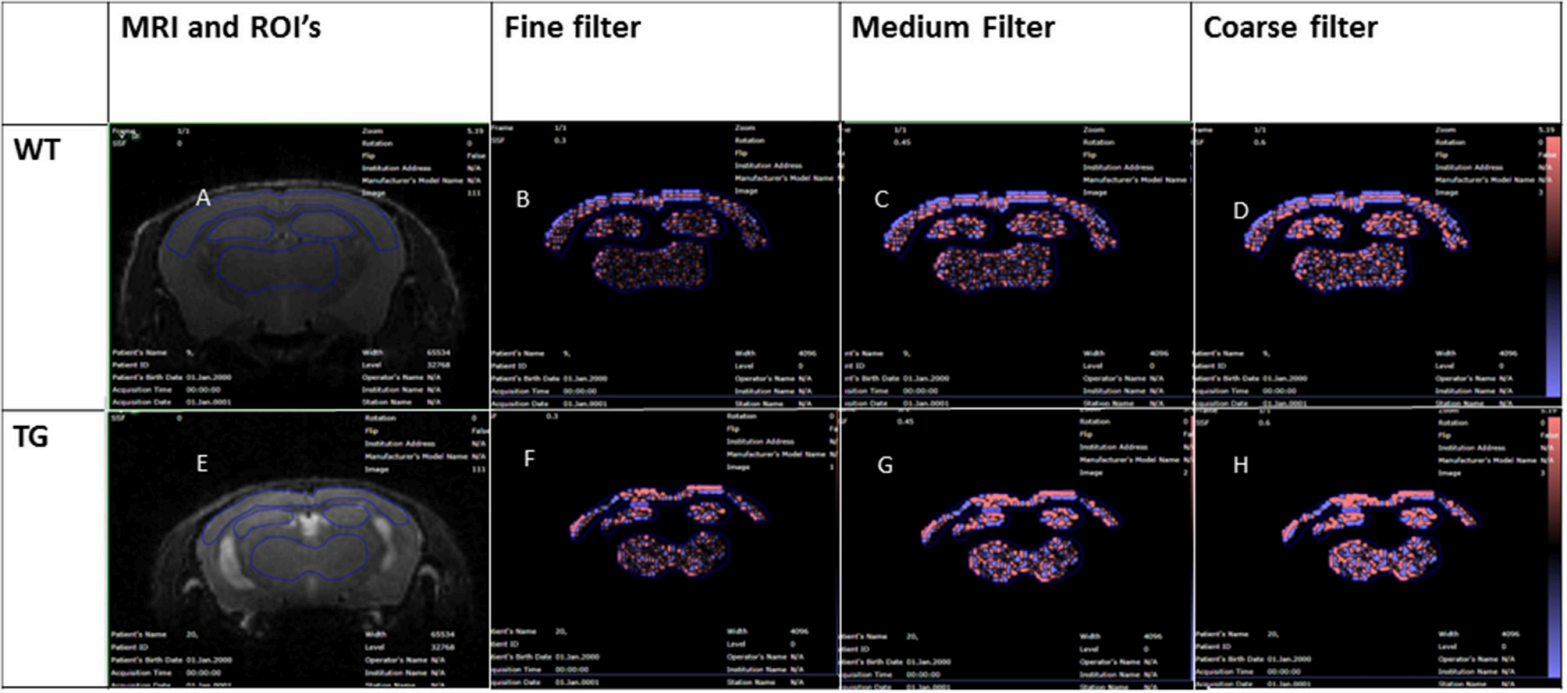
Wildtype and rTg4510 texture analysis images, MRI image with ROI's (A,E), fine filter images (B,F), medium filter (C,G), and coarse filter (D,H).
